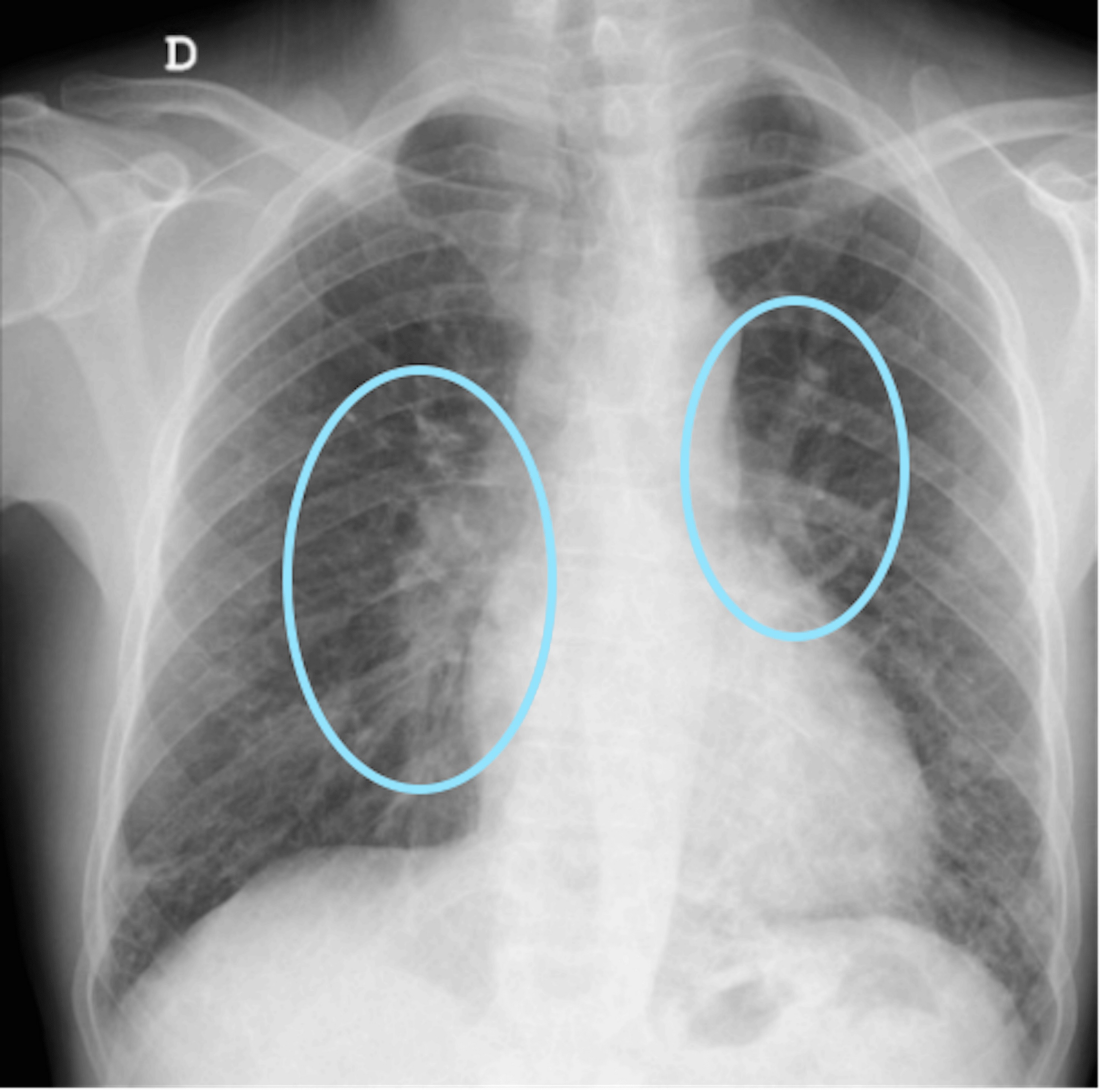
Follow-up chest X-ray one year after the acute episode, showing complete radiographic resolution of the previously observed bilateral perihilar opacities (highlighted areas).
